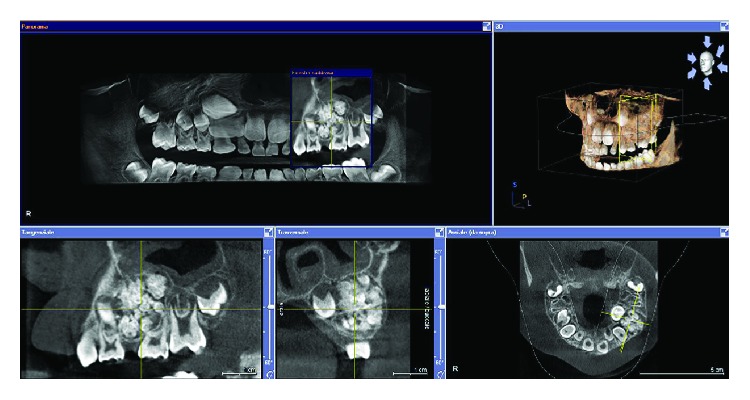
Cone-beam computed tomography showing radiopaque calcified structures with irregular margins characterized by a tooth-like configuration enclosed in radiolucent peripheral borders. The entire lesion measures approximately 12 × 15 × 19 mm.
